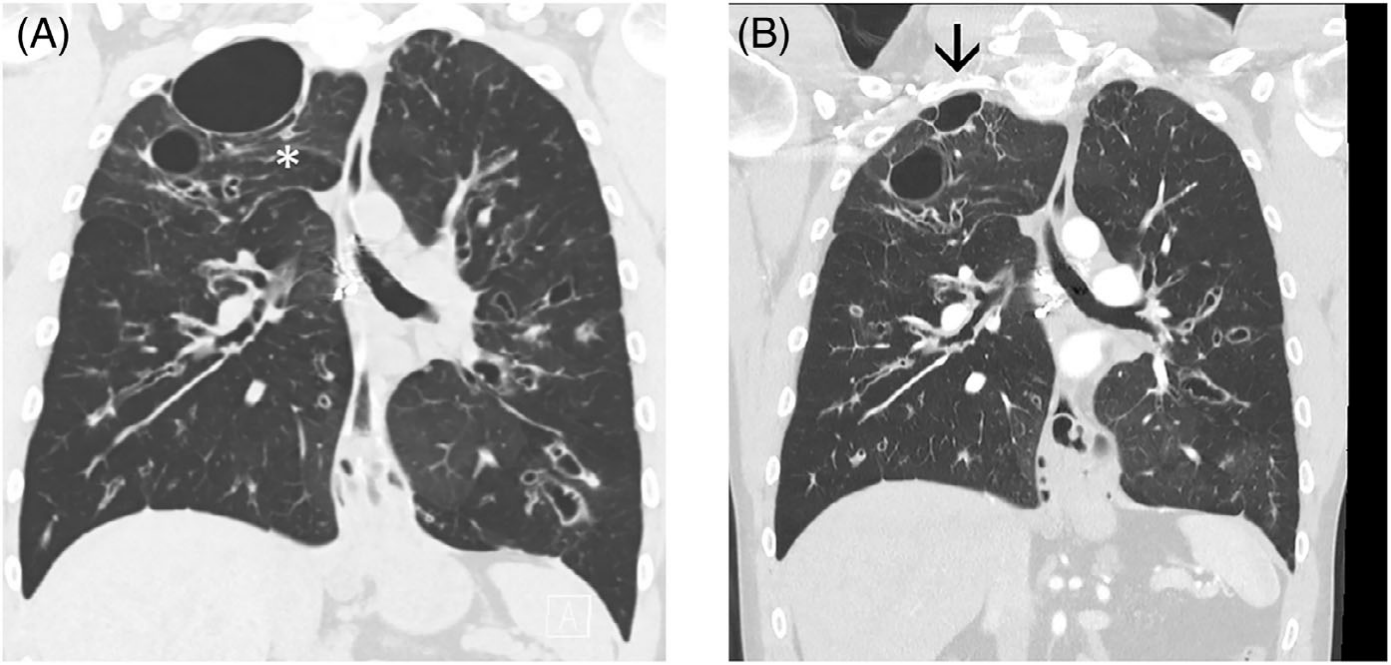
CT scans before (A) and after (B) elexacaftor + tezacaftor + ivacaftor for case 1
